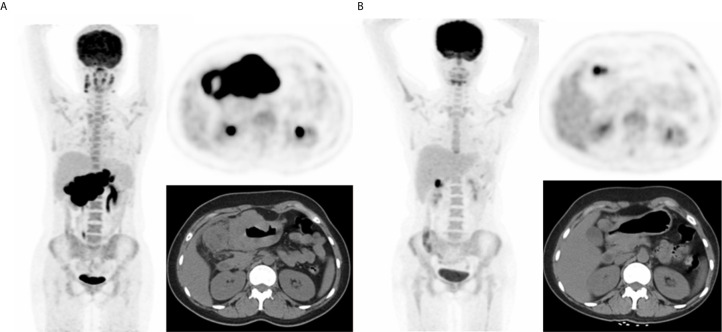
A representative case of a 25-year-old female with stage IIIA BL and both nodal and extranodal disease. Baseline maximum intensity projection (MIP) (A) showing hypermetabolic lesion in stomach and abdominal nodes. End of treatment PET/CT (B) showing high 18F-FDG uptake residual lesion in the lymph node of gastric, considering a partial response compared to baseline scan. This patient died nine months after baseline PET/CT.
